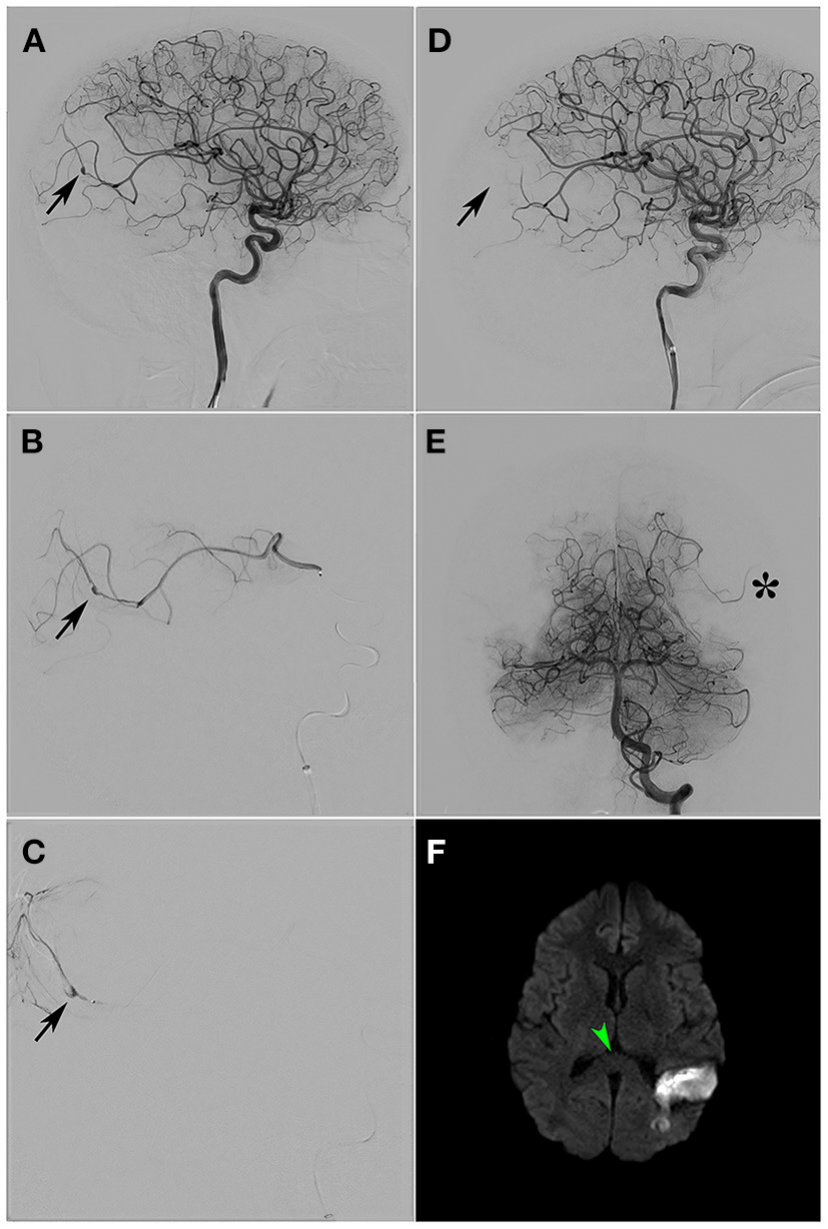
(A) Selective arteriography of RICA. The presence of a pseudoaneurysm is detected at the level of the distal temporo-occipital vasculature (black arrow). (B) Angular artery supraselective arteriography. The branch presenting the pseudoaneurysm shows an irregularity of the arterial wall suggesting arteritis (black arrow). (C) Glue cast after embolization (black arrow). (D) Selective arteriography of RICA after branch embolisation. Absence of pseudoaneurysm (black arrow). (E) Selective arteriography of LVA. Compensation of the left temporo-occipital territory through temporal branches of the left vertebral artery (black asterisk) is demonstrated. (F) Diffusion weighted MR image 1 month after treatment. Morphological and signal normalization of the splenium of the corpus callosum (green arrowhead). Absence of additional ischemic lesion after embolisation. RICA, Right internal carotid artery; LVA, Left vertebral artery.
